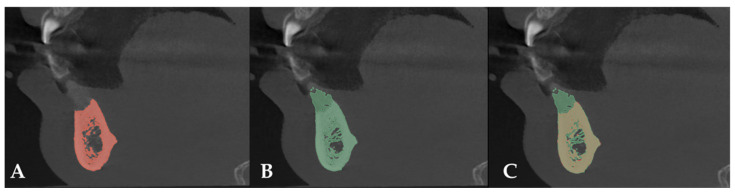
(A) Preoperative CT scan. (B) Postoperative CT scan. (C) Superimposition of preoperative and postoperative CT scans.
